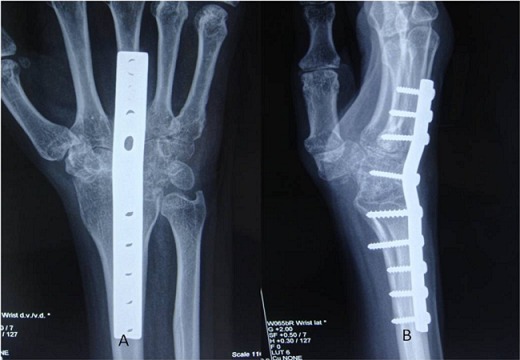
AP (a) and Lateral (b) radiographs showing sound union at one year follow up
